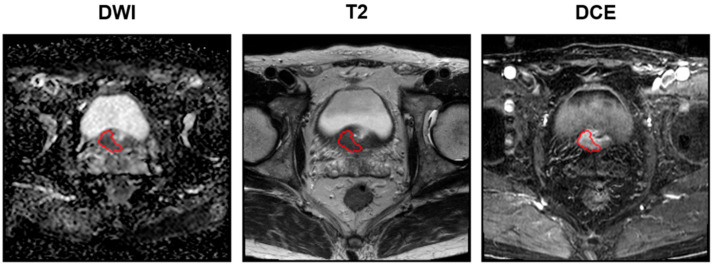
Examples of delineation on mpMRIs: DWI (left), T2 (middle), and DCE (right) sequences. The red circles denote the tumor ROIs. Images acquired on a Philips 3T Scanner. Scan magnification scale: ×1. The patient’s information: post-RP BCR (+), initial PSA of 13.32 mg/dL, mpMRI: suspicious ECE (cT3a), surgical pathology findings: GS of 9 (4 + 5), ECE (pT3a), no lymph node involvement (pN0), PSM. mpMRI = multiparametric magnetic resonance imaging; DWI = diffusion-weighted image; T = Tesla; DCE = dynamic contrast-enhanced; RP = radical prostatectomy; BCR = biochemical recurrence; PCa = prostate cancer; GS = Gleason score; ECE = extracapsular extension; PSM = positive surgical margin.
